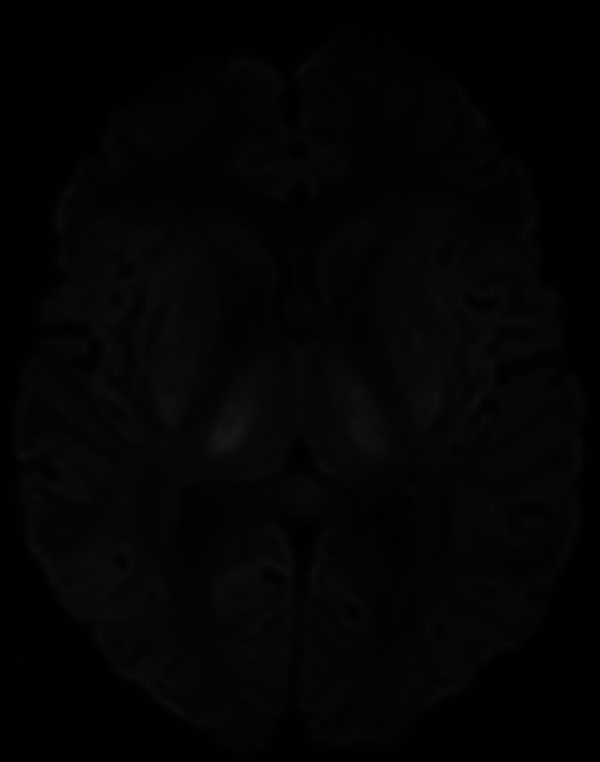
Axial B1000 DWI image showing slightly higher signal in the lentiform nuclei and laterally in the thalami.
